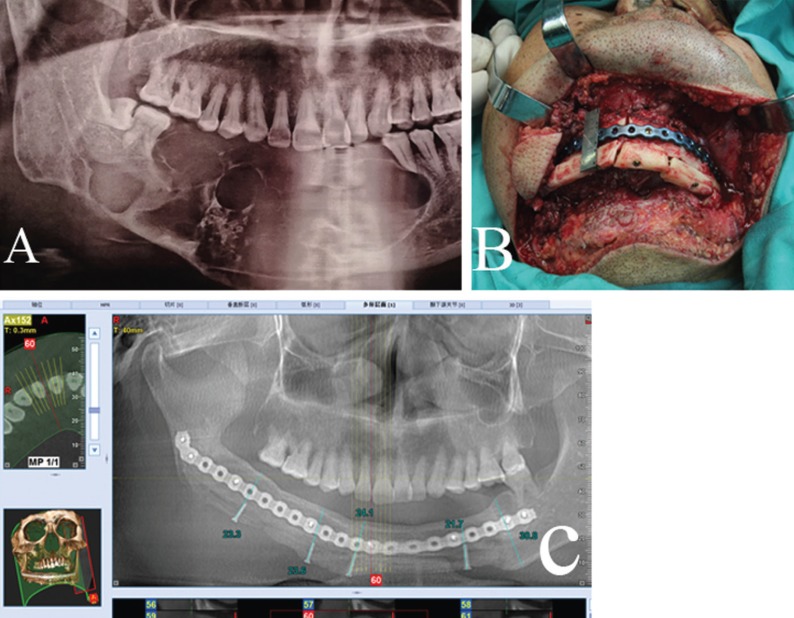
A. Preoperative panoramic radiograph showed a mandibular ameloblastoma. B. The nonvascularized fibular flap has been sagittalized and segmented, make a small groove at the lower edge, and then fix it to the upper vascularized fibular flap with a 2.0×10.0mm titanium screw. C. Postoperative CBCT showed the vascularized and nonvascularized fibular flap survived well 14 months later.
